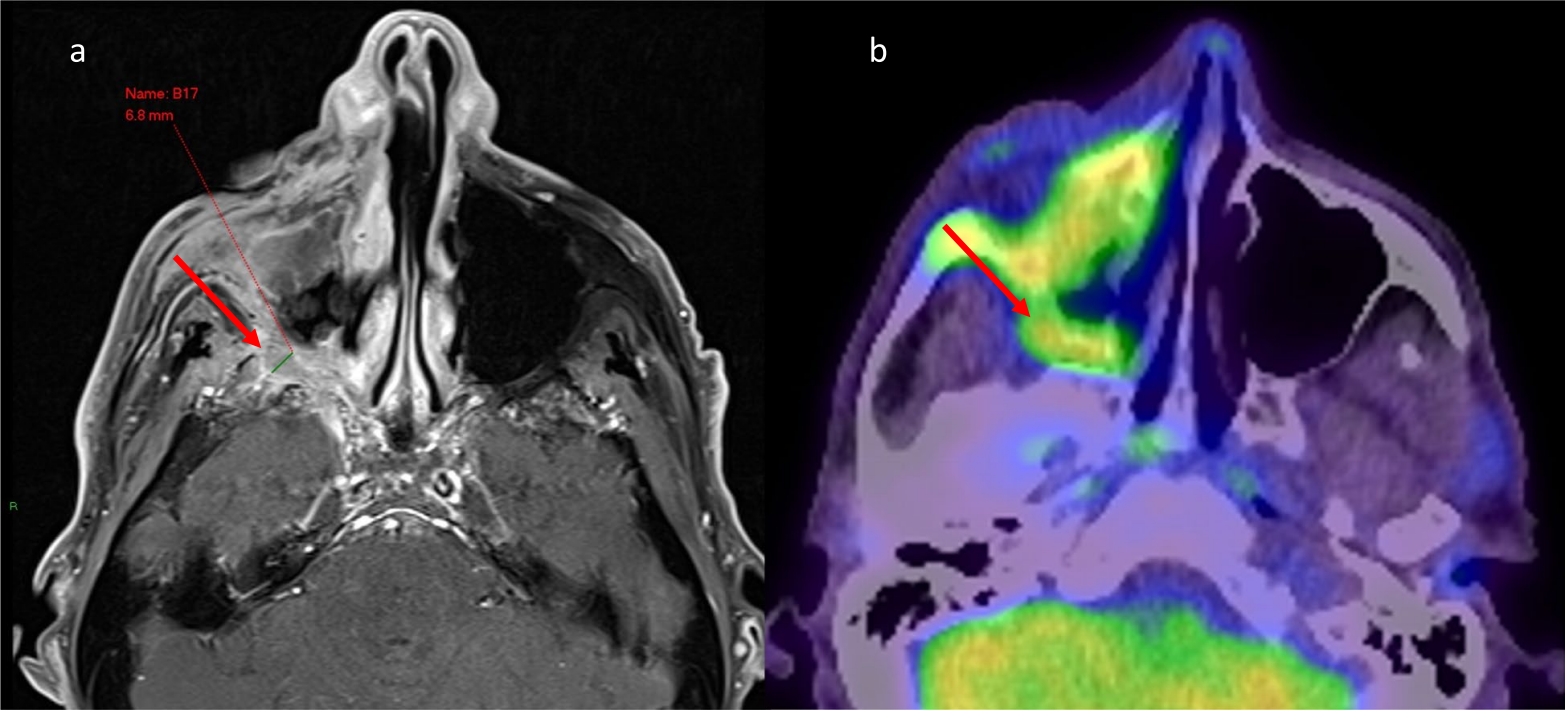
Perineural spread (PNS) demonstrated on fluorodeoxyglucose-positron emission tomography computer tomography (FDG-PET/CT), concordant with magnetic resonance imaging (MRI): Large right facial cutaneous squamous cell carcinoma (CSCC) with extensive perineural spread. A Axial post-contrast T1 fat saturation (FS) MRI demonstrates thickening of the right infraorbital nerve, with thickness 6-7 mm, in the right pterygopalatine fossa, right inferior orbital fissure and right infraorbital canal. This is concordant with (B) curvilinear FDG avidity on FDG-PET/CT in the right pterygopalatine fossa, right inferior orbital fissure and right infraorbital canal
